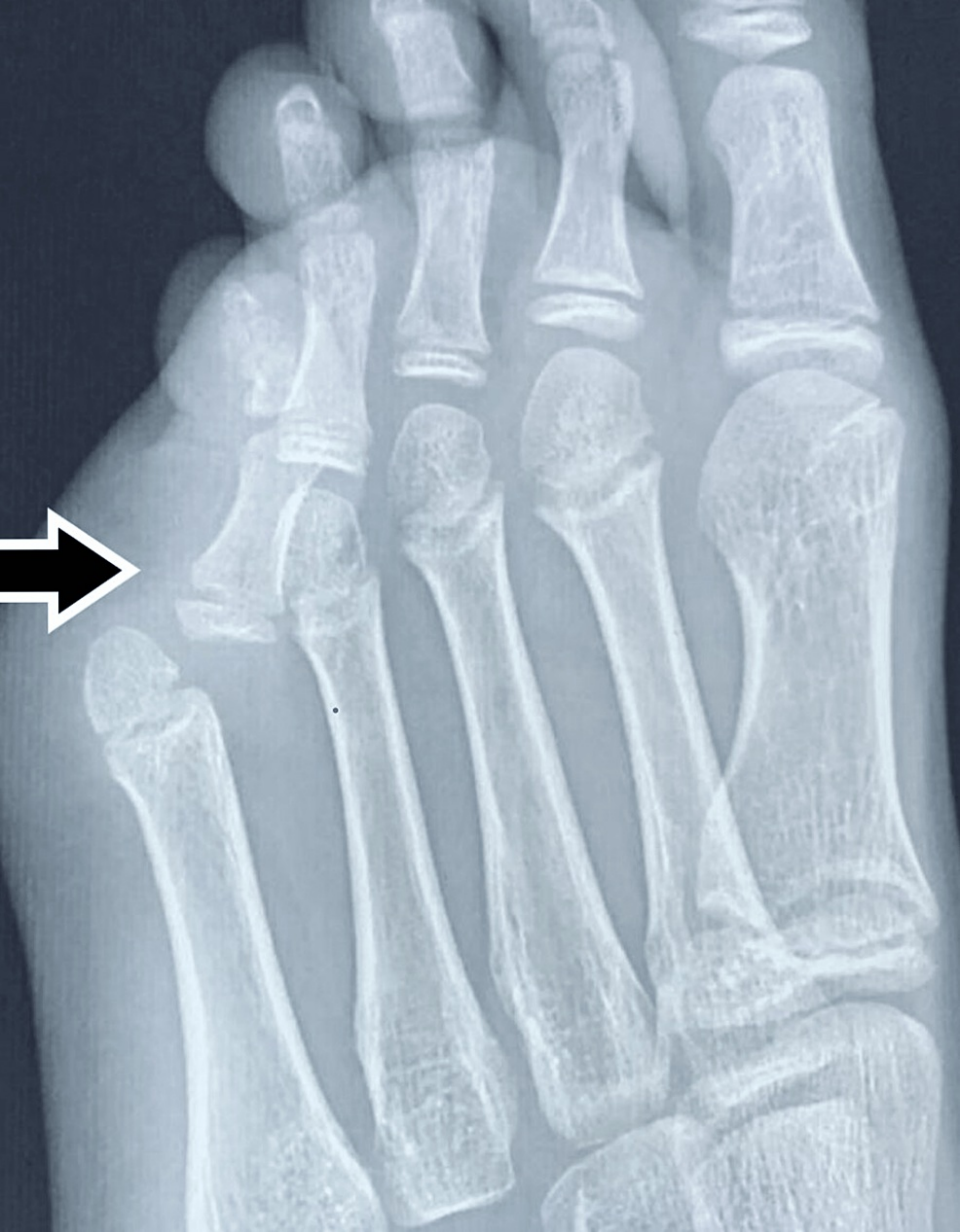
Pre-operative (at the time of presentation, two years after injury) x-ray showing dislocation of the fifth metatarsophalangeal joint
